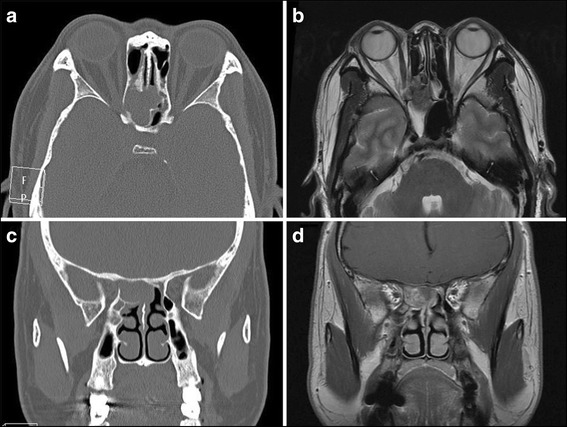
Neuroimaging for case 1, male, 47y, chronic sphenoid sinusitis (right, VA = 20/200). a: Axial computed tomography scan shows bony destruction at the right orbital apex. b: Axial, T2-weighted magnetic resonance imaging scan reveals a homogenous, hyperintense lesion of the inferior aspect of the right sphenoid sinus. c: Coronal computed tomography scan demonstrating erosion of the bony medial orbital apex. d: Coronal, T1-weighted, contrast-enhanced magnetic resonance imaging scan without contrast shows heterogenous signal in the right sphenoid sinus
